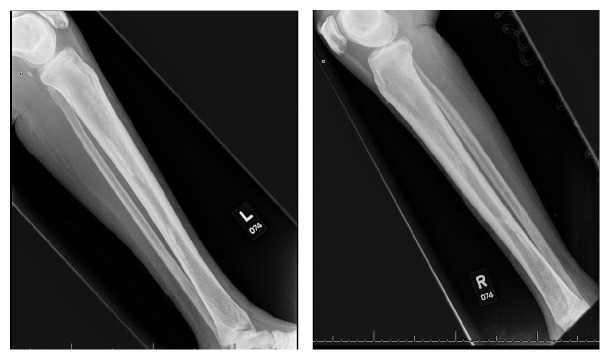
X-ray of both legs showing regions of sclerotic cortical thickening identified in the posterior distal metadiaphysis and anterior metadiaphysis of the tibia with additional sclerosis of the metadiaphyseal regions of the fibula.
